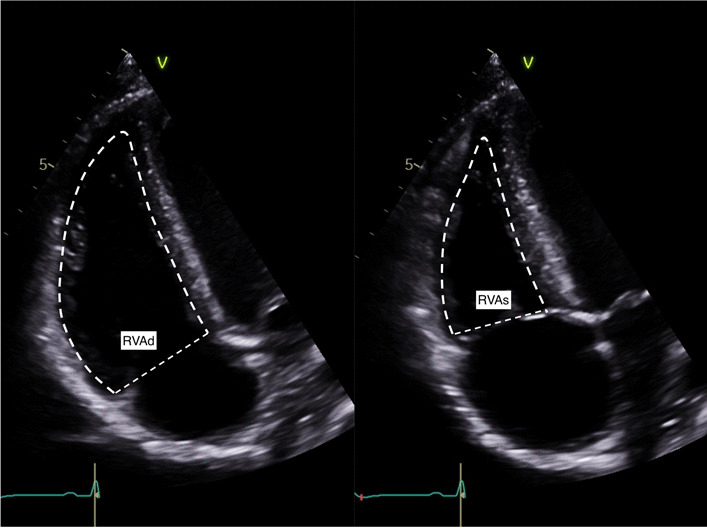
RV pulsed tissue Doppler S Wave (S’) velocity	PW tissue Doppler S wave measurement taken at the lateral tricuspid annulus in diastole. It is important to ensure the basal RV free wall segment and the lateral tricuspid annulus are aligned with the Doppler cursor to avoid velocity underestimation
